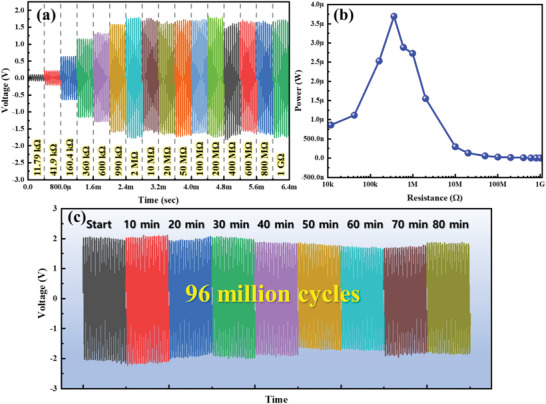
Parametric study of ESING as a function of various factors. Load resistance‐dependent a) output voltage and b) output power of ESING. The ultrasound intensity was 74 dB, and the gap between the PVDF membrane and the Al electrode was 9 mm. c) Durability data measuring the output voltage of ESING during operation for 80 min measured every 10 min.
